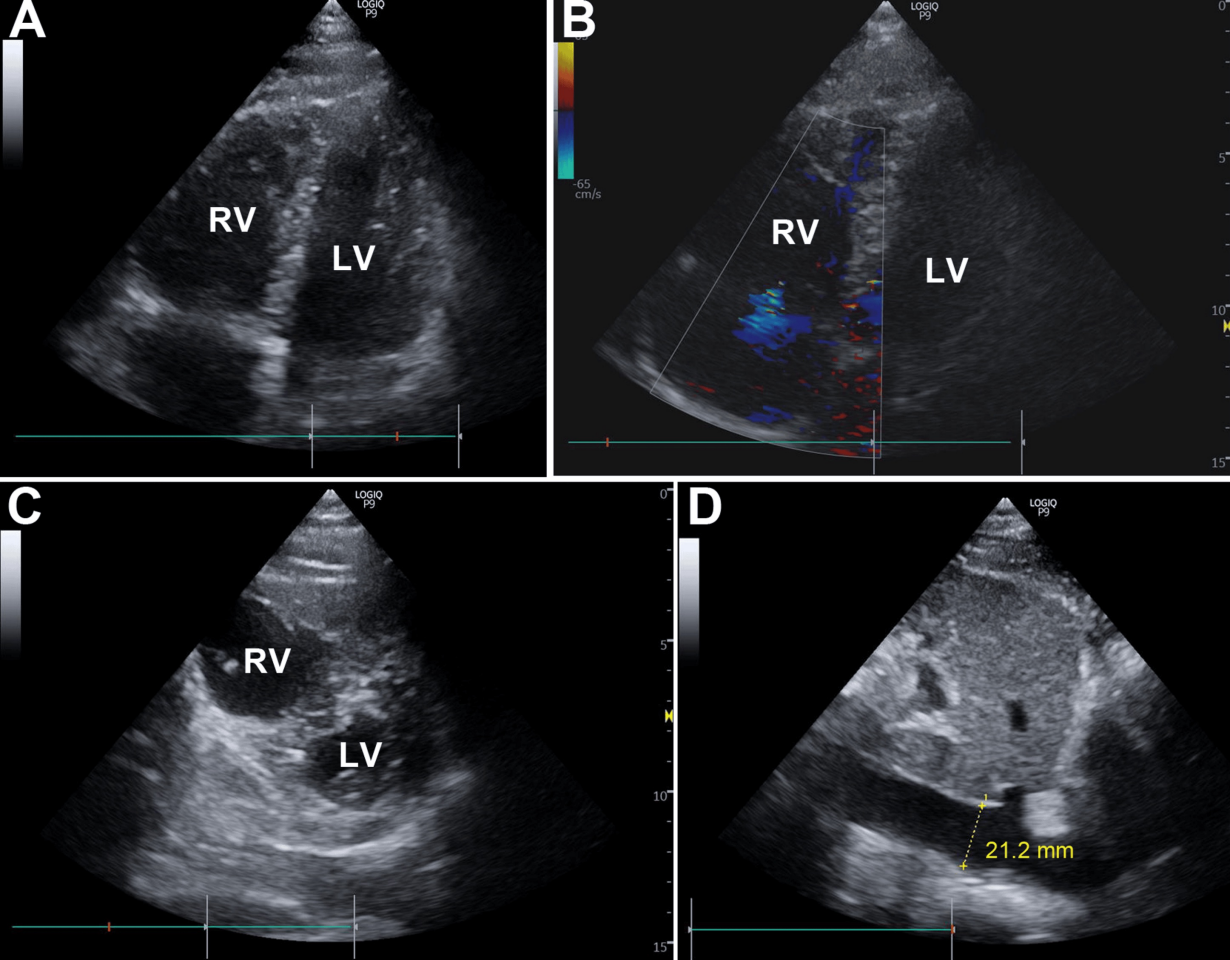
Echocardiography on admission Echocardiography demonstrates an enlarged RV (A), mild tricuspid regurgitation (B), D-sign formation in the LV (C), and poor respiratory variation of the inferior vena cava (D). LV, left ventricle; RV, right ventricle
